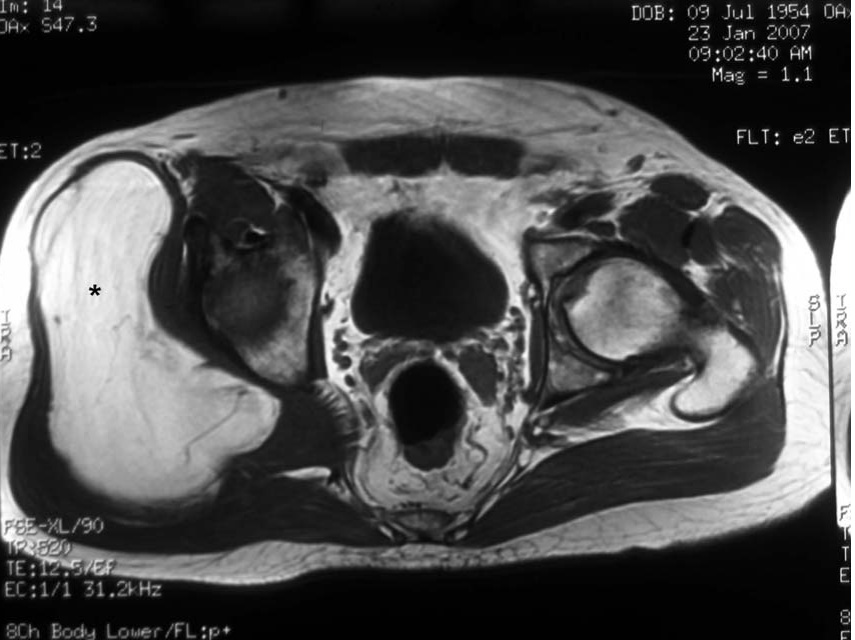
Magnetic resonance imaging showing the giant lipoma (*) underneath the right gluteus maximus.
